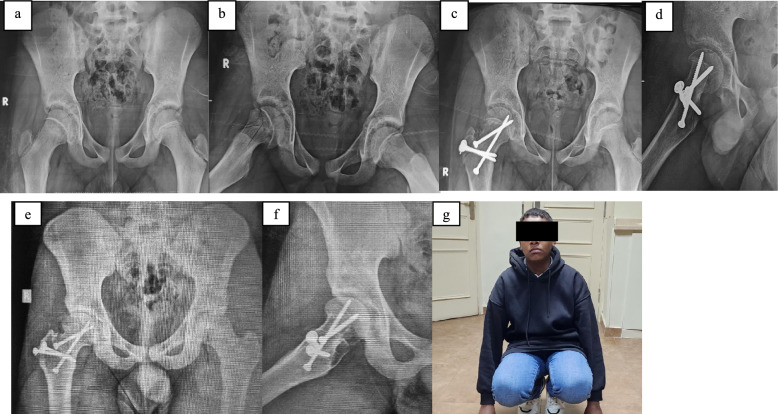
a, b Preoperative radiographs (ap and lateral) showing right SCFE in 12-year-old boy. c, d Postoperative radiographs (ap and lateral) follow-up at 1 year follow-up after MDP and fixation by two cannulated fully threaded 4 mm screws. e–g Postoperative radiographs (ap and lateral) at the final follow-up visit (7 years) and photo showing excellent functional outcome with equal hip flexion range on both sides
